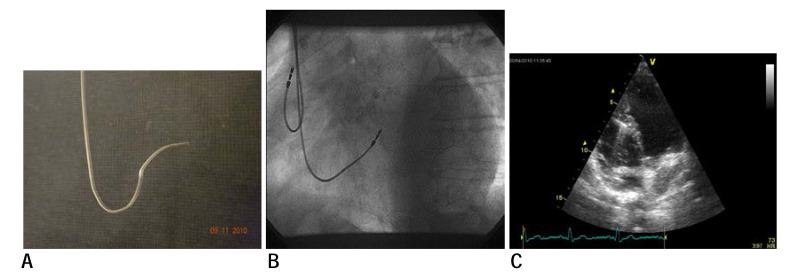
A- double curved stilet used for RV septal lead placement; B- LAO fluoroscopic incidence of a septal RV lead; C- apical 4 chamber view showing septal placement of RV lead.
